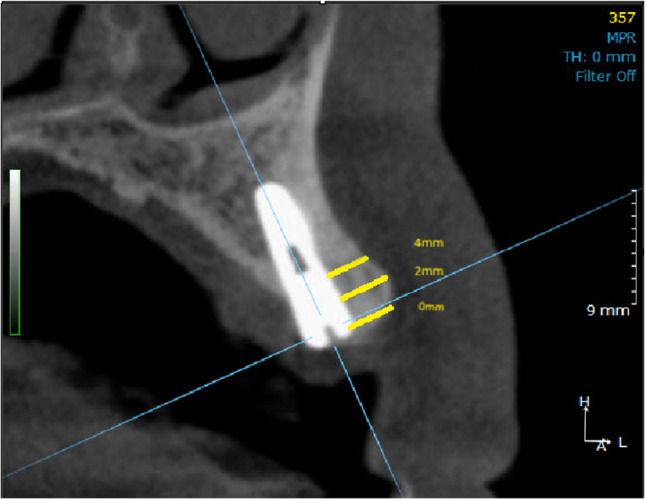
Measurement of BBT. A cross sectional CBCT image showing measurement of BBT, the implant was utilized as a reference for the measurement of BBT from the cross-sectional view by adjusting panoramic long axis in its center and bisecting it (showing the buccolingual dimensions) represented by blue lines. BBT was measured horizontally from the implant surface to the outer buccal bone plate at three different levels vertically represented by yellow lines 0 mm from implant crest, 2 mm below implant crest, and 4 mm below implant crest immediately after surgery
